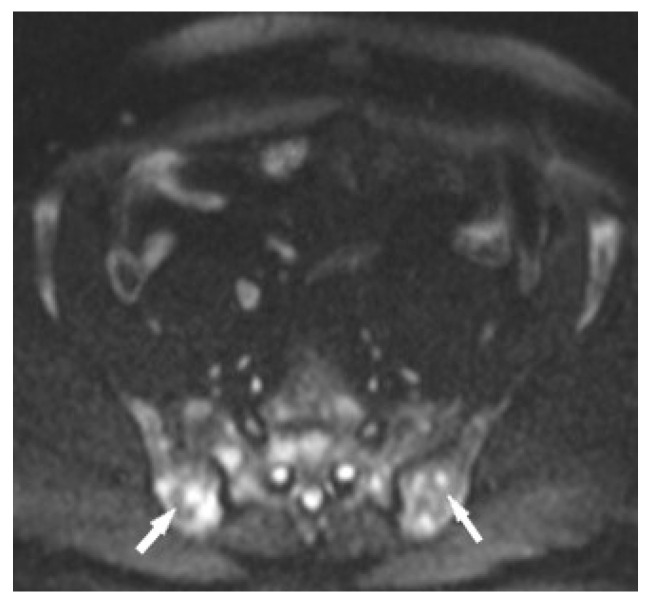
MRI of the pelvis, Diffusion-Weighted Imaging (DWI). Multiple bone marrow lesions with restricted diffusion (white arrows).
